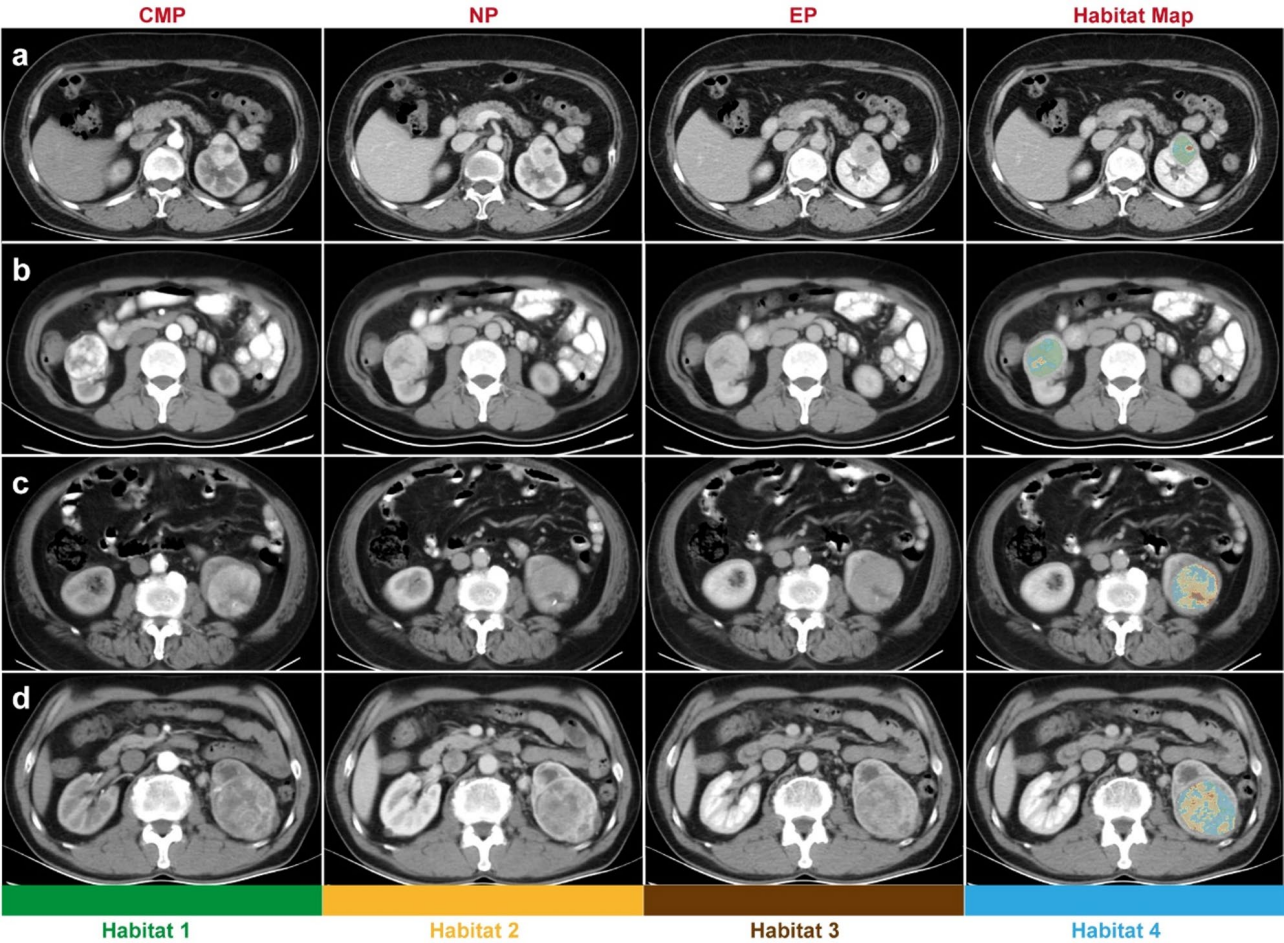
Representative CT images of two low-grade ccRCCs (a, b) and two high-grade ccRCCs (c, d). Note: (1) Tumor habitat maps overlaid on EP-phase images. (2) Each color represents a distinct tumor habitat
